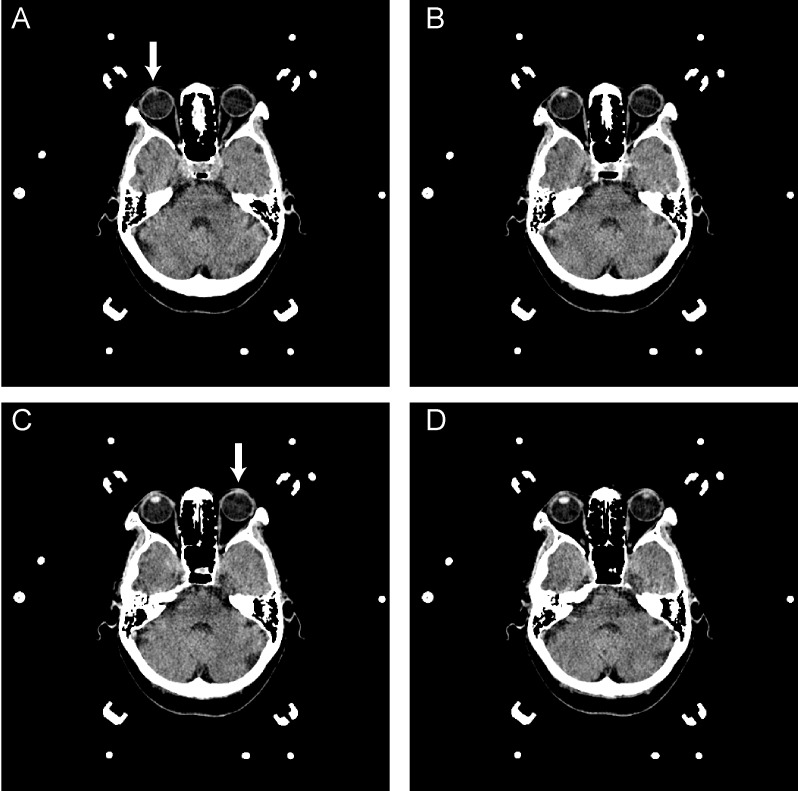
A–D are serial axial images of a brain CT for DBS surgery. The interval distance of the serial image is 1 mm. A reveals the upper edge of the right lens (arrow); however, the left lens was unavailable in this image. In C, the upper edge of the left lens was presented, as indicated by the arrowhead. The vertical deviation was calculated from the serial image as 2 mm.
